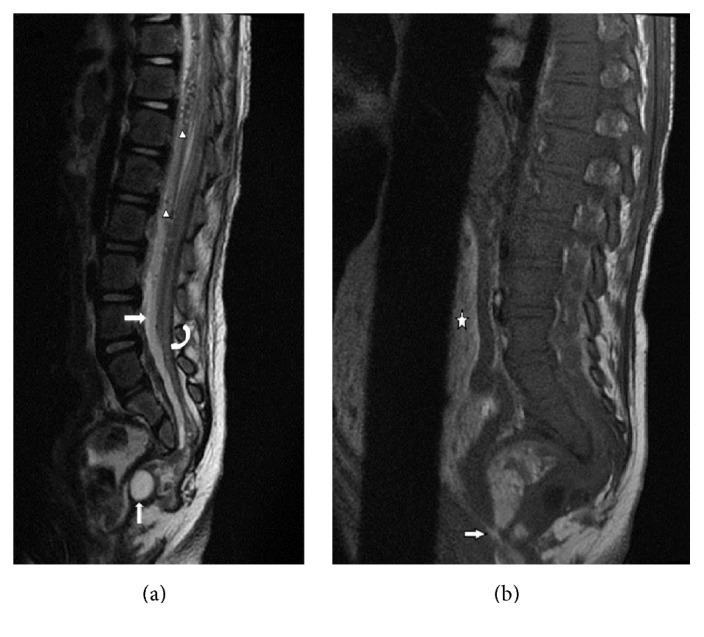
Spine MRI on day 5 to further characterize the presacral mass. (a) Sagittal T2 weighted MR image of the lower spine shows complex presacral mass with a small separate hyperintense cyst communicating with the thecal sac, which could be meningocele (thin arrow). Spinal canal shows epidural fluid collection (arrow) with multiple air foci (arrowheads). There is low-lying conus and a tethered cord, reported in 70% of Currarino syndrome cases (curved arrow). (b) Sagittal T1 weighted MR image of the spine shows the communication of the larger presacral mass with the anal canal (arrow). The contents of the mass have similar intensity to the contents of the dilated sigmoid colon (asterix).
